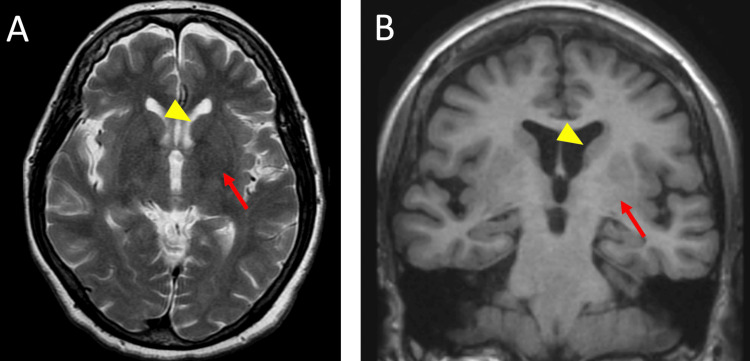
Brain magnetic resonance imaging (A) T2-weighted and (B) T1-weighted images do not show caudate nucleus atrophy or hemorrhagic and ischemic lesions in the basal ganglia. In (A) and (B), the yellow arrowhead indicates the caudate nucleus and the red arrow marks the lentiform nucleus (comprising the putamen and the globus pallidus).
